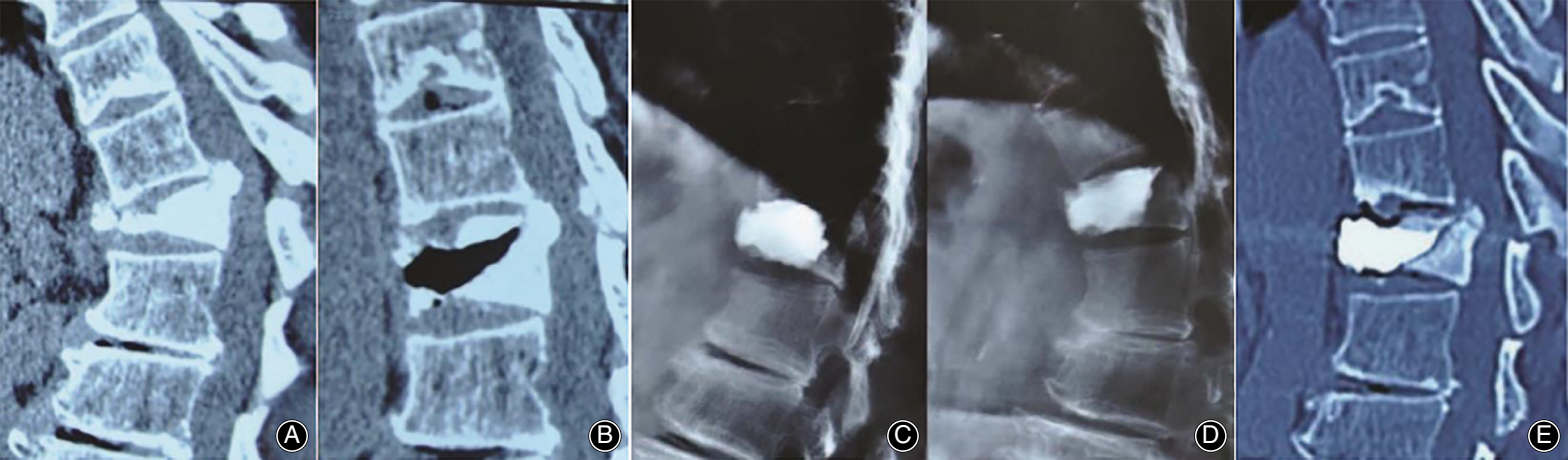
A typical patient with pattern II IVC from Zhang *et al*.^4^. (A) Preoperative hyperflexion lateral CT. (B) Preoperative hyperextension lateral CT. (C) and (D) Postoperative dynamic x‐ray shows the twelfth thoracic vertebra (T12) vertebra stability. (E) CT revealed the dislocation of cement one month after the PKP.
